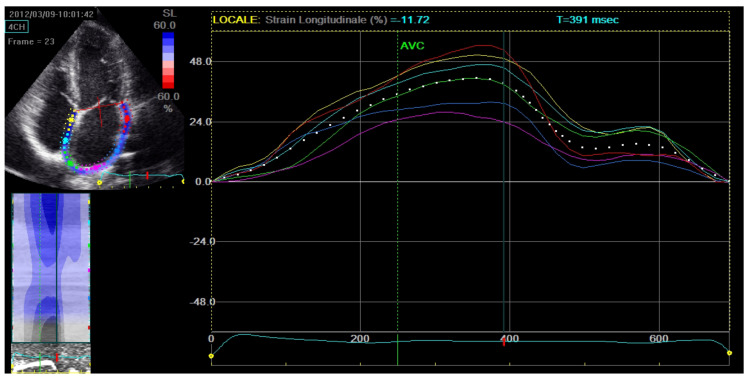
LA in a 4-chambers view divided into six segments by the STE software, each providing its own strain value. Top: the curves of the individual segments’ strain and the average curve, with the first peak representing PALS and the second peak PACS. LA: left atrium; STE: speckle tracking echocardiography, PALS: peak atrial longitudinal strain; PACS: peak atrial contraction strain.
